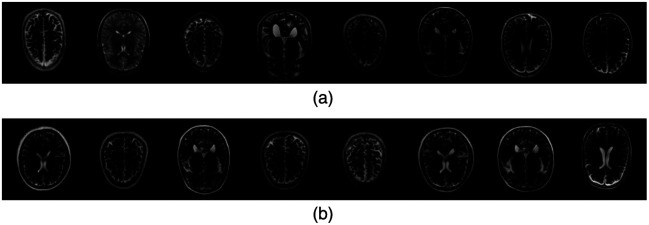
Comparison of real and GAN-generated MRI images. The GAN produced visually plausible images. (a) Real: clinical MRI images. (b) Fake: MRI images generated by the GAN.
